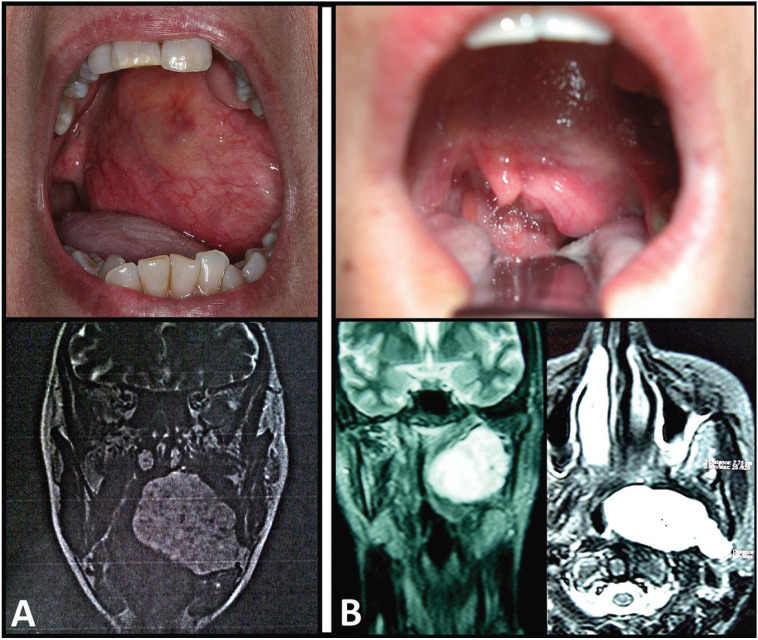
Preoperative clinical view of both patients. A. Intraoral and coronal MRI views of case 1. The pleomorphic adenoma located in the left parapharyngeal space encompasses the entire left soft palate. The tongue is constricted and the uvula is displaced to the right. B. Intraoral, coronal and axial MRI views of case 2.
